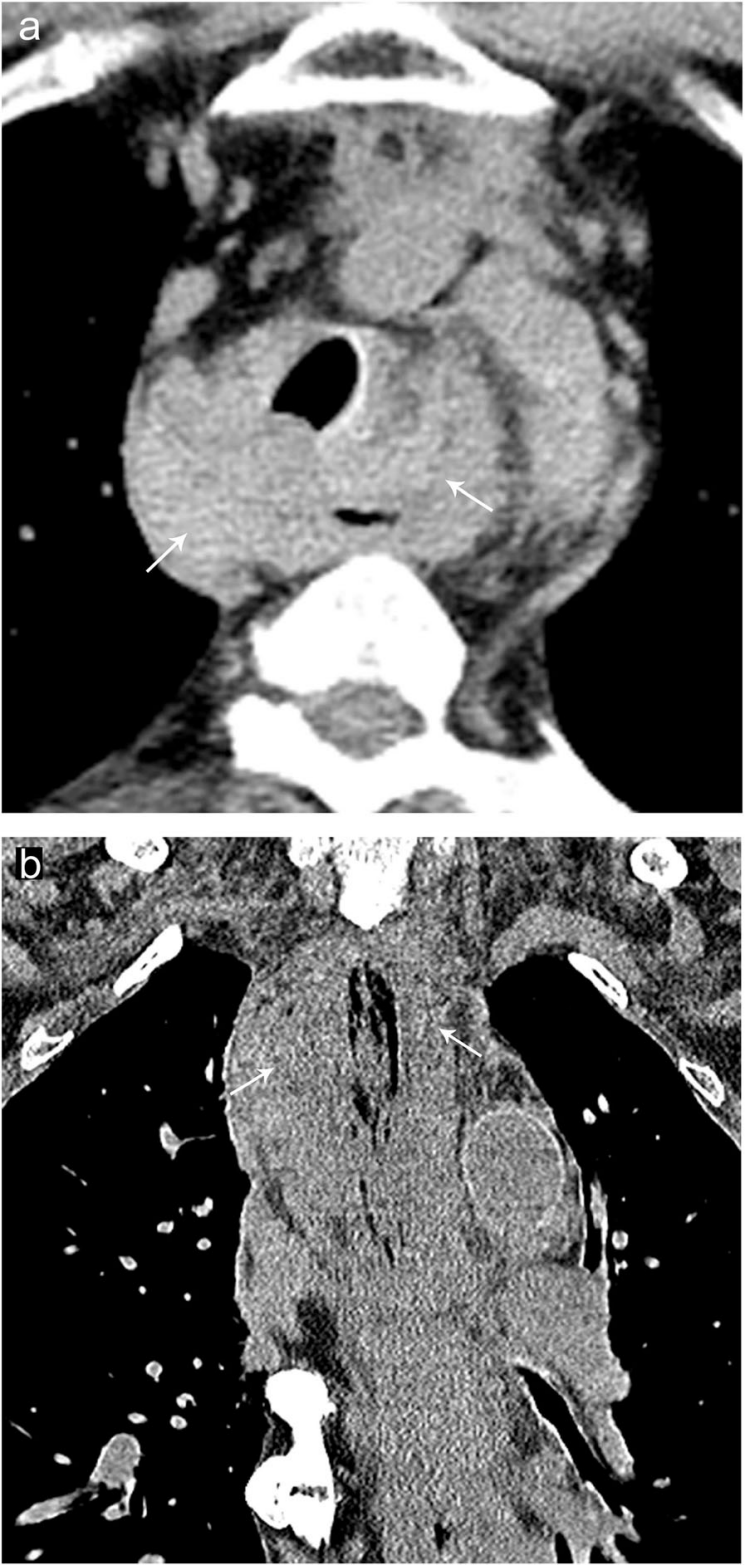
Mediastinitis. Axial (a) and coronal (b) noncontrast CT images demonstrating inflammatory changes and soft tissue stranding (arrows) of the mediastinum secondary to mediastinitis. Note the associated diffuse thickening of the esophageal wall in this patient with suspected esophageal wall perforation
